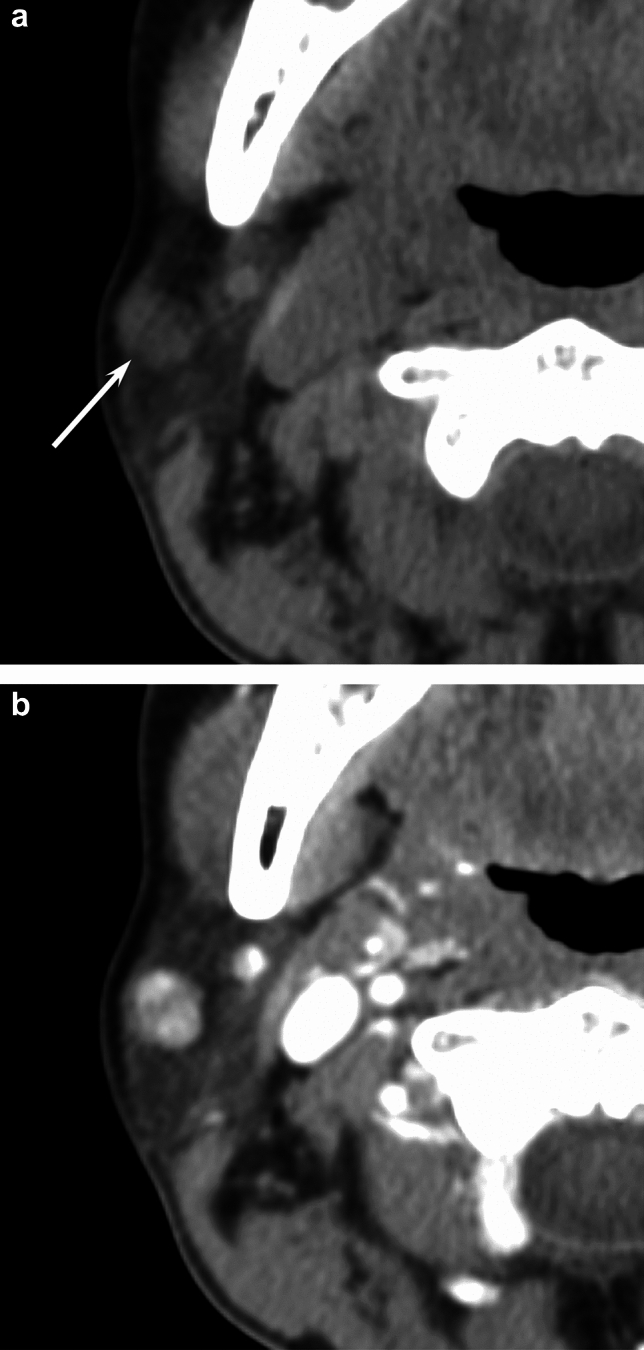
A 52-year-old woman with myoepithelioma of the right parotid gland. a Unenhanced CT image shows a hypodense lesion (arrow) (12 HU). b Contrast-enhanced CT image shows strong enhancement (104 HU)
